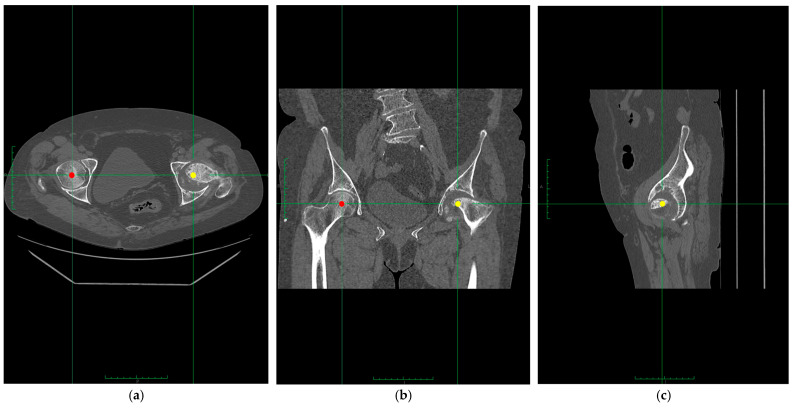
Anterior center edge angle was measured using multiplanar CT reconstruction to accurately determine the center of the acetabulum. Axial (a), coronal (b), and sagittal (c) planes were correlated to establish the acetabular center. In both axial and coronal planes, the healthy contralateral femoral head ((a,b)—red dot) was used as a reference point, positioning the axes (green line) through the center of the healthy femoral head. By correlating these two planes ((a,b)—yellow dot), the acetabular center was determined in the sagittal plane, thus allowing ACEA measurement in the absence/resorbed femoral head.
